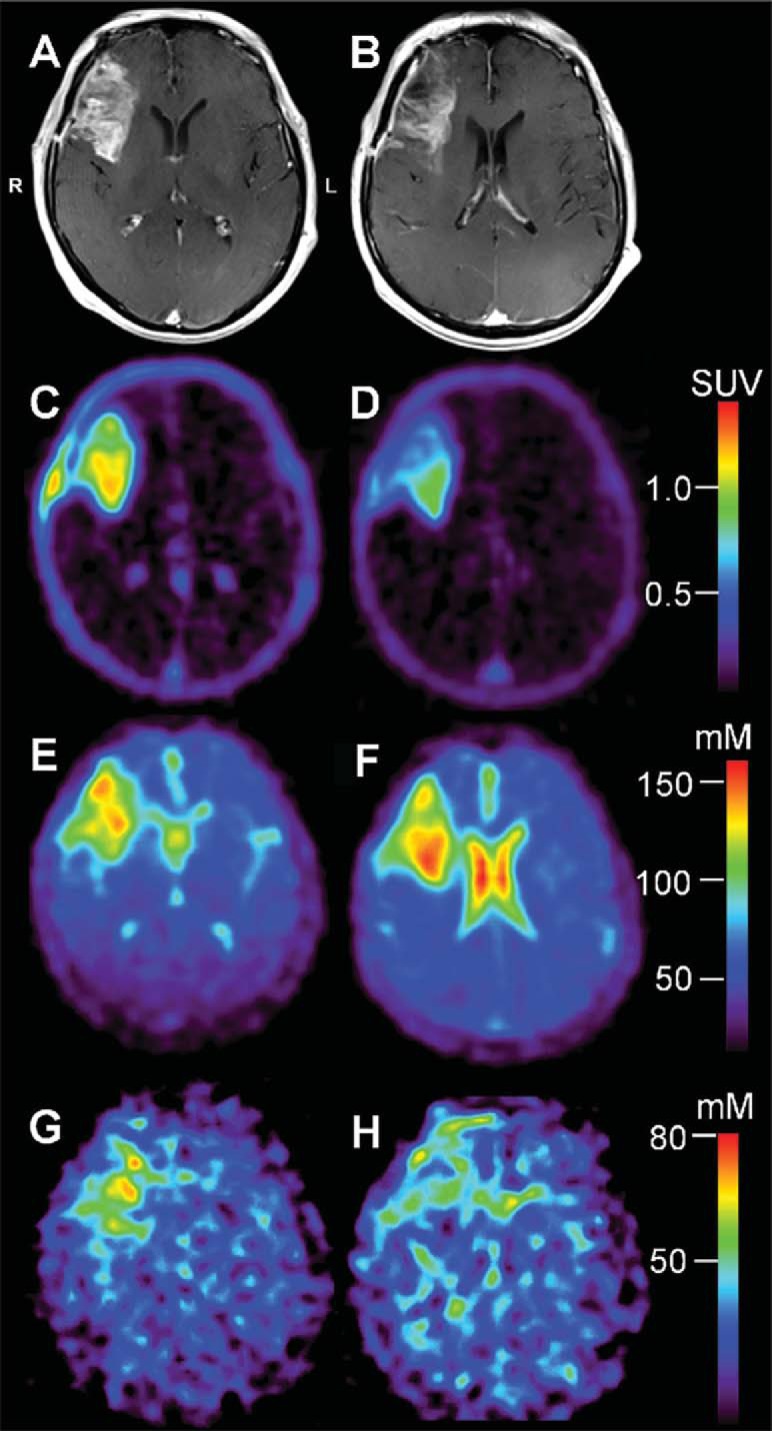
Patient ML-10 #4. Representative baseline (BL; before therapy initiation) and early-therapy assessment (ETA; 15 days after therapy initiation) contrast enhanced MRI (CE-MRI; top row, A and B, respectively), BL and ETA [^18^F]ML-10 PET (second row, C and D, respectively), BL and ETA total sodium concentration (TSC) MRI (third row, E and F, respectively), and volume-fraction-weighted bound sodium concentration MRI (vBSC-MRI; bott'm row, G and H, respectively) sections of a 56-year-old male with a new GBM diagnosis. All images are shown coregistered to the patient's BL CE-MRI. Due to a technical challenge, this subject's ETA PET was acquired 55 min later than scheduled, meaning that the imaging time frame reflects 175–205 min post injection, instead of 120–150 min post-injection, which was the protocol. At BL, the patient's CE-MRI scan (A) shows a large, peripherally enhancing and centrally necrotic intra-axial mass centered in the right frontal lobe extending to the floor of the anterior cranial fossa and involving the medial–anterior aspect of the right insula, and the right aspect of the genu of corpus. The ETA CE-MRI (B) shows a decrease in the size and contrast enhancement with anterior and central necrosis. Overall, this represents an excellent response to therapy. On PET, BL [^18^F]ML-10 SUV image (C) shows intense therapy-naive uptake (SUV_max_ = 1.41), particularly in the posterior portion of the mass. Compared with BL, the ETA [^18^F]ML-10 SUV image (D) shows a decrease in the uptake (SUV_max_ = 1.07) consistent with very low apoptosis in the anterior portion of the mass, and significant reduction in the posterior portion of the mass. At BL, the patient's TSC-MRI (E) shows high ^23^Na concentration in the CSF portion of the tumor, positioned anteriorly, with moderately increased (compared with contralesional hemisphere) TSC, posteriorly, in the viable tumor tissue. Similarly, the BL vBSC image (G) shows increased signal (compared with contralesional hemisphere) in the viable tumor tissue component. At ETA, the TSC-MRI (F) shows an increase in TSC at the region of the viable tumor tissue, with a corresponding decrease in vBSC (H) in the same location, suggesting that the increased TSC is a result of increased free sodium due to response to therapy, consistent with the patient's long time-to-progression (18 months).
